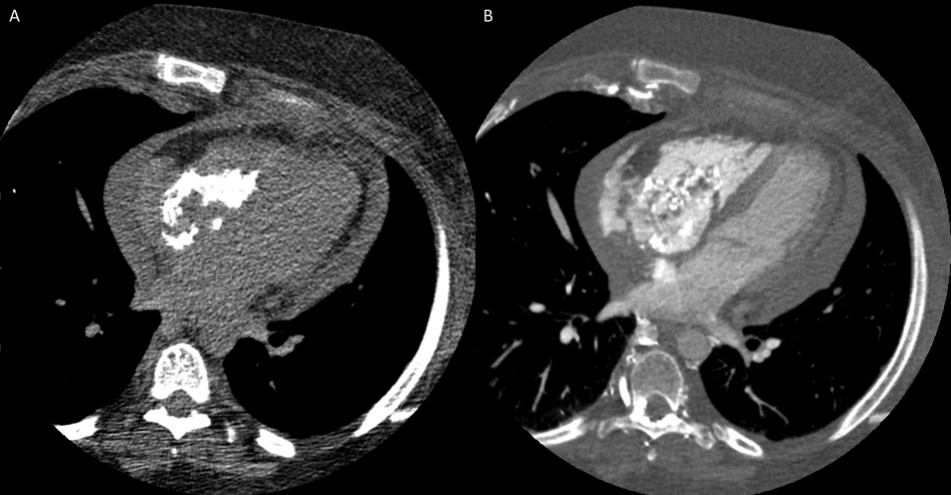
CT heart morphology revealed a heterogeneous irregular calcified mass extending into the right atrium, across the tricuspid valve. This mass had characteristics suggestive of an organized chronic thrombus, as webs of thrombus were extending throughout the right atrium and almost completely occluding the distal superior vena cava. A small pericardial effusion is also present.
